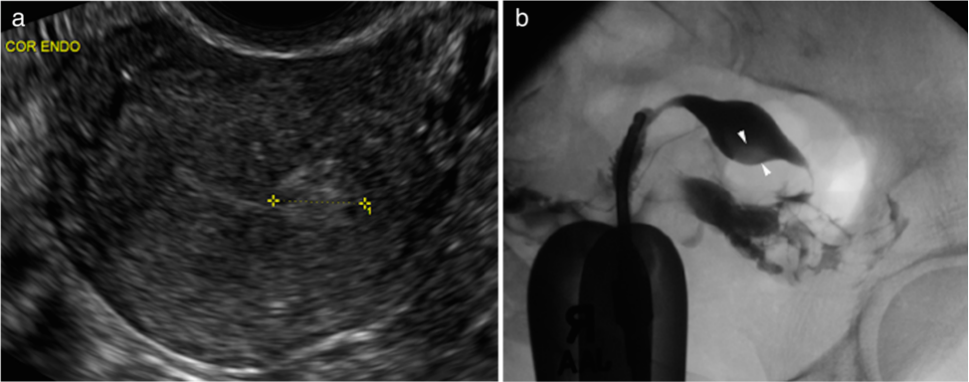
a 44 year-old G0P0 female with inability to conceive for 4 years presents for baseline assessment prior to IVF. TVS demonstrates an 11 × 11 × 10 mm echogenic lesion within the left aspect of the endometrial cavity (calipers). Flow was demonstrated within the lesion with color Doppler, raising the possibility of endometrial polyp. b Corresponding HSG demonstrates a depended rounded filling defect within the left aspect of the endometrial cavity, which persisted on multiple projections, suggestive of a polyp. The fallopian tubes are normal in caliber and patent. The patient went on to HSC, where the lesion proved to be a submucosal fibroid
